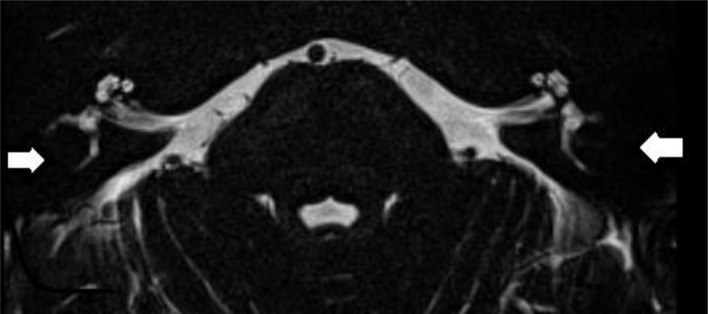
Axial T2-weighted turbo spin echo MRI of the left and right labyrinth demonstrating signal loss in the lateral semicircular canal (arrow) corresponding to the axial CT slice in Figure 1.
